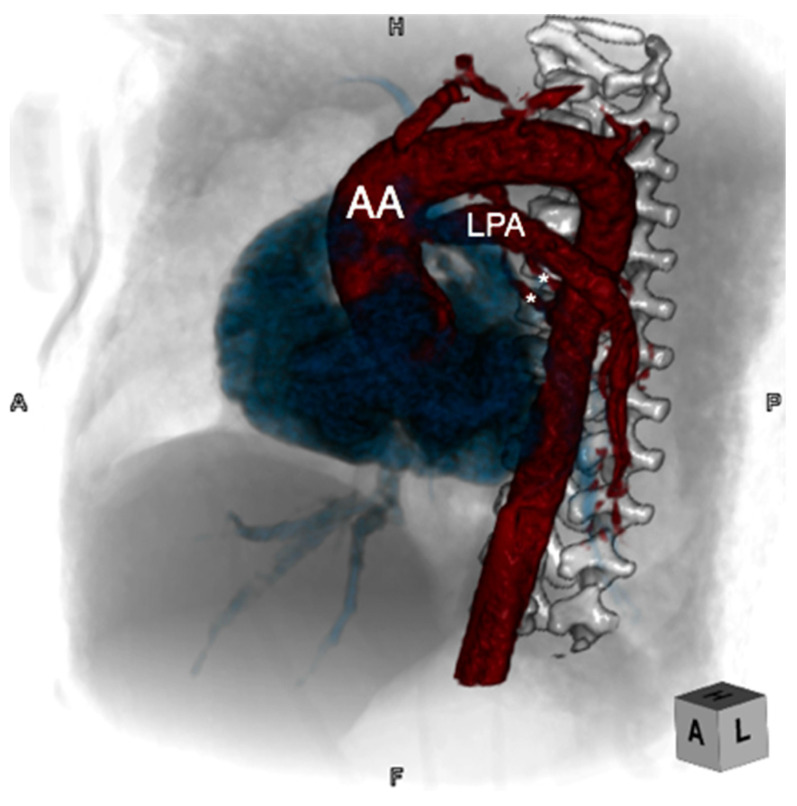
Computed tomographic three-dimensional (3D) reconstruction angulated in a left anterior oblique plane demonstrating an anomalous left pulmonary artery (LPA) arising from the ascending aorta (AA) in a patient with tetralogy of Fallot. Two small major aortopulmonary collateral arteries (asterisks) are demonstrating arising from the proximal thoracic descending aorta and coursing to the right lung.
